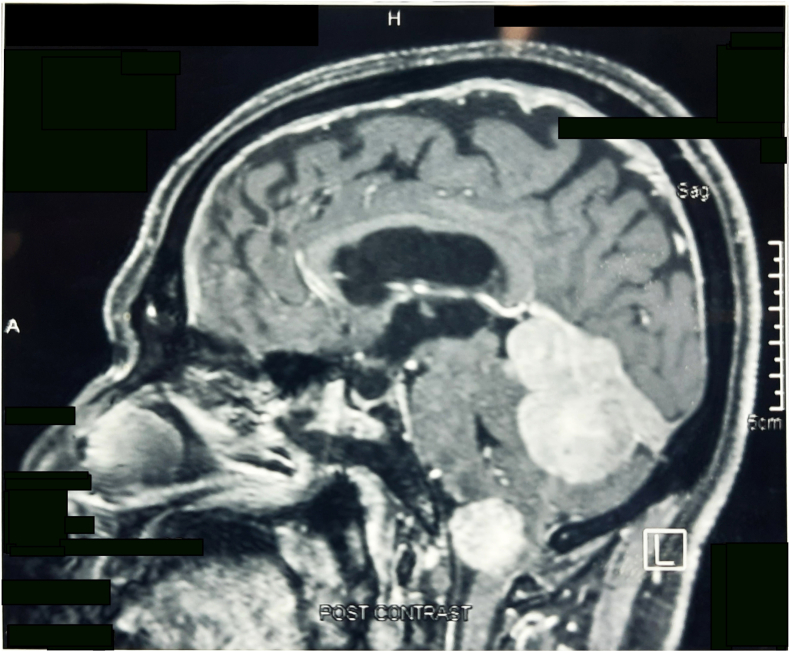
MRI Brain shows two separate extra-axial lesions in the posterior aspect.
